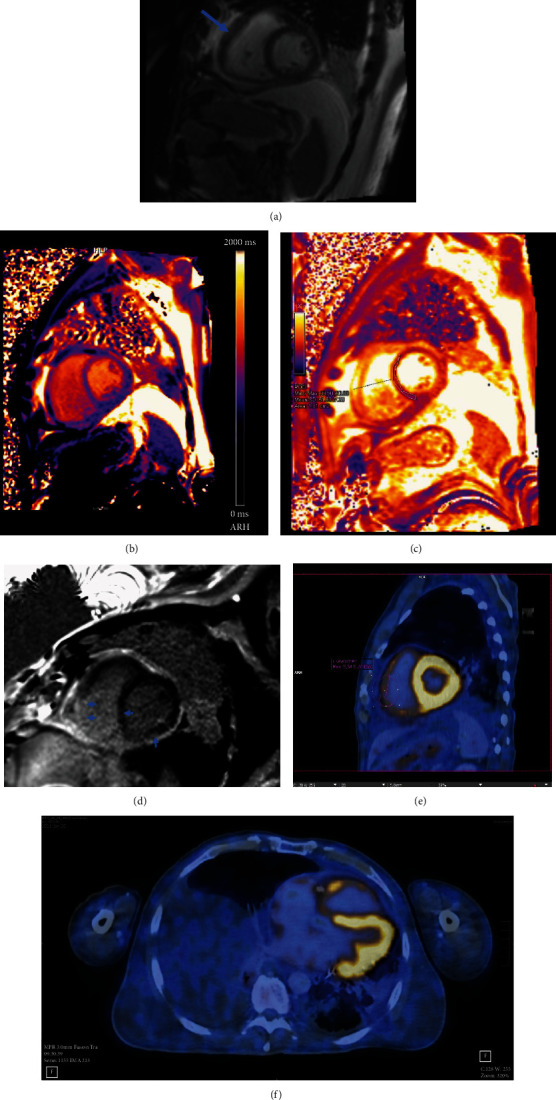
Cross-sectional studies confirmed myocardial inflammation. (a–d) Cardiac magnetic resonance imaging showing right ventricular hypertrophy (a, SSFP cine, ∗), increased T1 relaxation time (septal: 1062 ms, LV posterior wall: 1118 ms) and ECV of 33% (b, T1 map), increased T2 relaxation time to 52 ms septal and 60 ms in LV posterior wall (c, T2 map) and right ventricular, diffuse septal and left ventricular posterior wall late gadolinium enhancement (c, PSIR LGE, arrows) indicating myocarditis and diffuse biventricular fibrosis. (e, f) Positron emission tomography with computed tomography showing FDG uptake in the right ventricular myocardium (SUVmax 5.3), left ventricular uptake was within normal limits.
